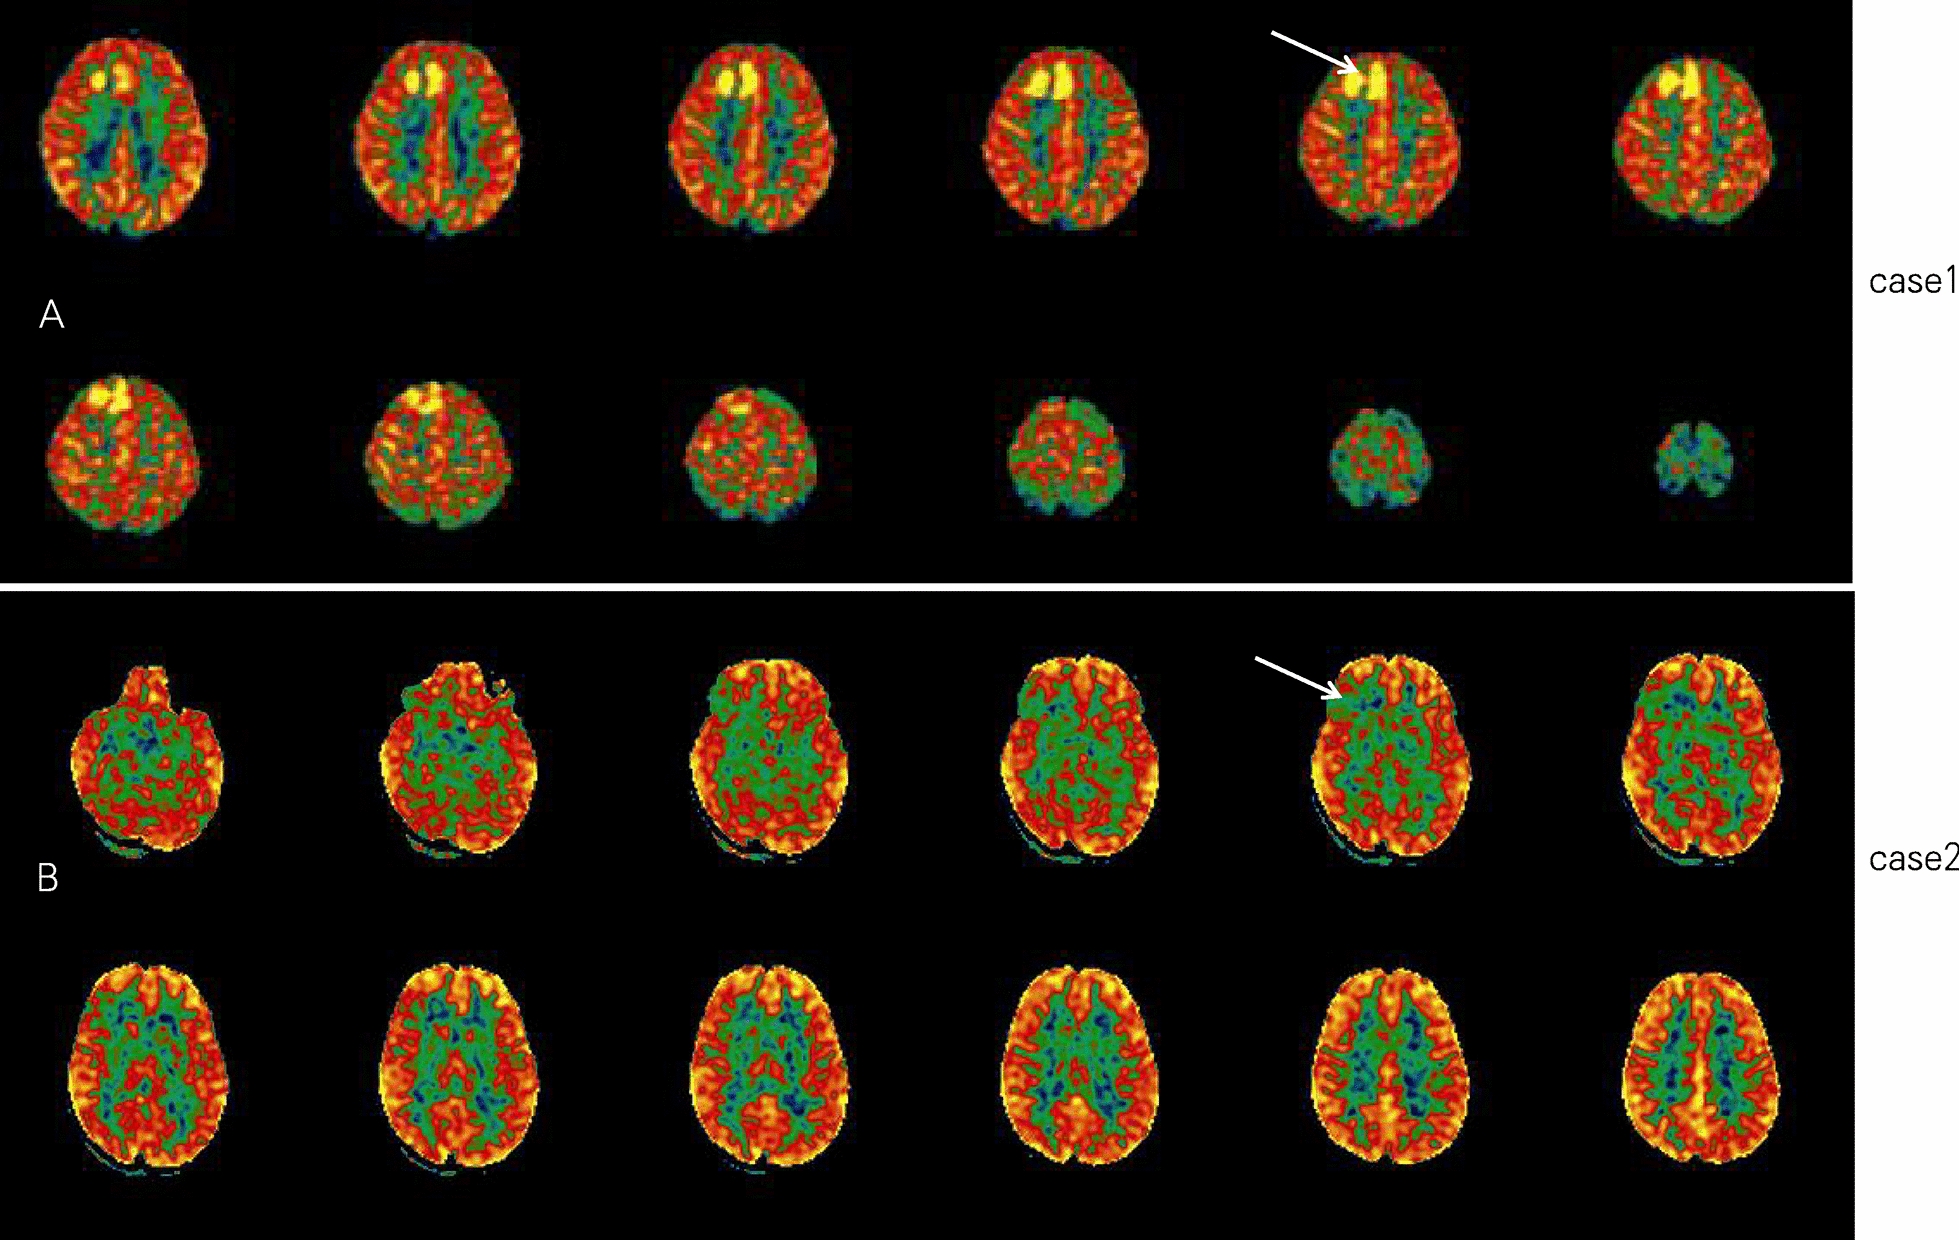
Preoperative ASL. A Case 1: ASL perfusion MRI depicts two hyperperfusions in the right medial frontal lobe, which is consistent with the epileptiform discharge position detected by VEEG and the suspicious thickened areas in FLAIR sequences. B Case 2: hypoperfusion was observed in the right frontal cortex, and the difference between the left and right frontal lobes exceeded the normal range of 0.2
